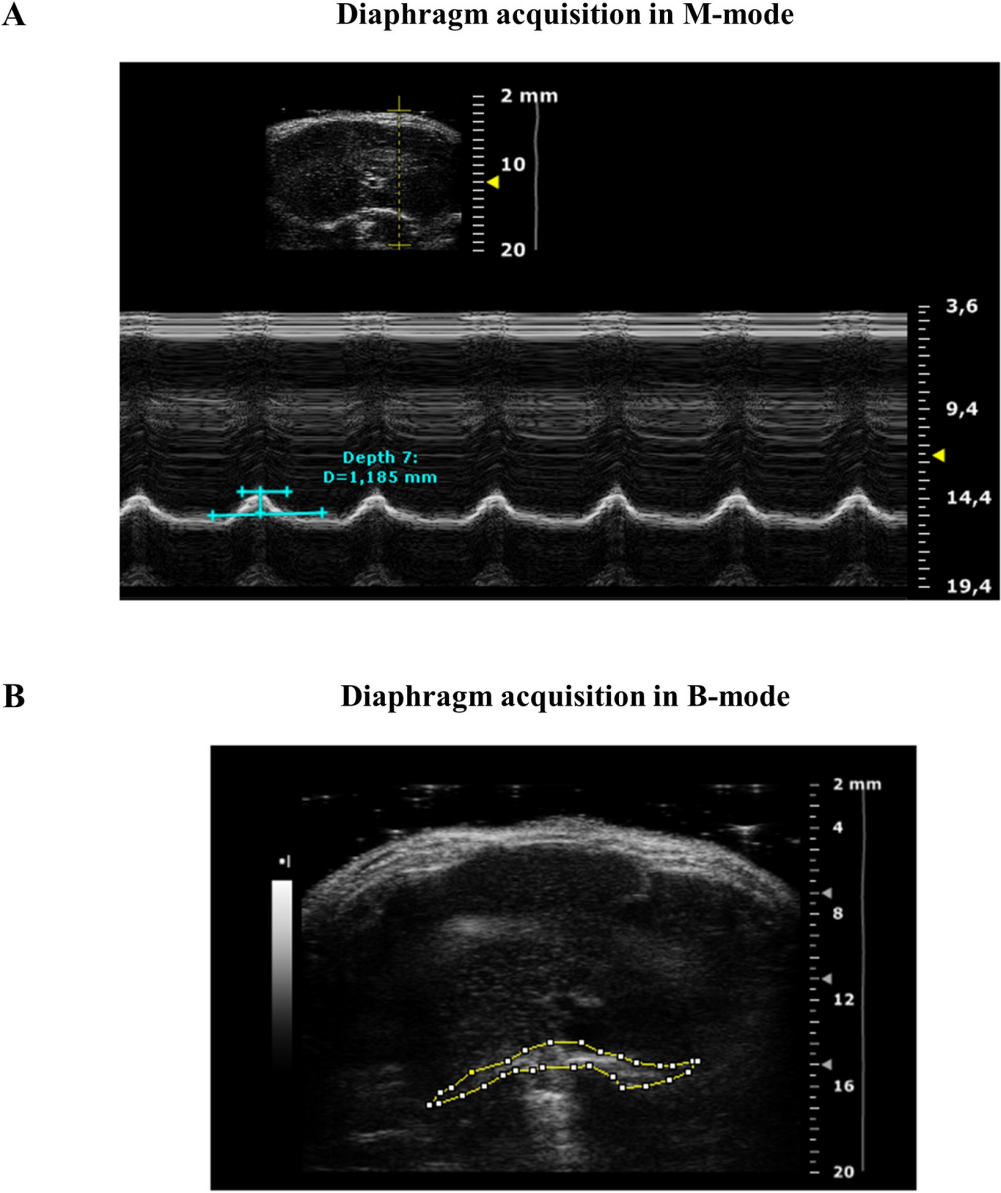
Sample images of ultrasonography diaphragm acquisition. The figure shows representative frames of the diaphragm acquired in M-mode (A) and B-mode (B), by ultrasonography. Diaphragm amplitude was measured in M-mode as the distance in mm, directly provided by the Vevo2100 software, between the baseline and the peak of contraction. The B-mode acquisition was used to perform echodensity analysis performed by using the Image J software. Mean pixel echodensity was obtained from the analysis of the outlined diaphragm section of constant dimensions of 4514.0 ± 17.6 pixels.
